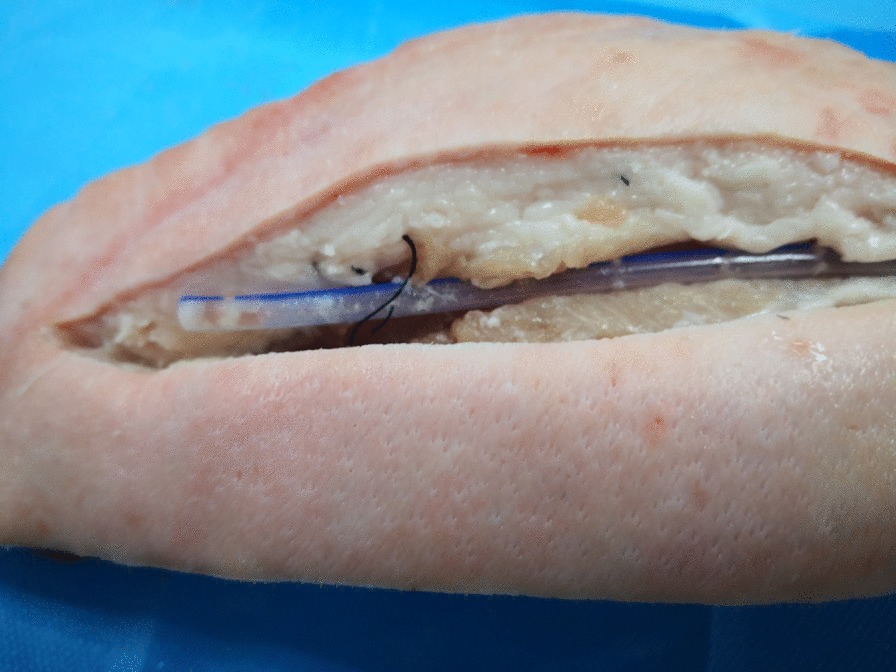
The sutured site of the drain located by ultrasound was confirmed by reopening the incision
